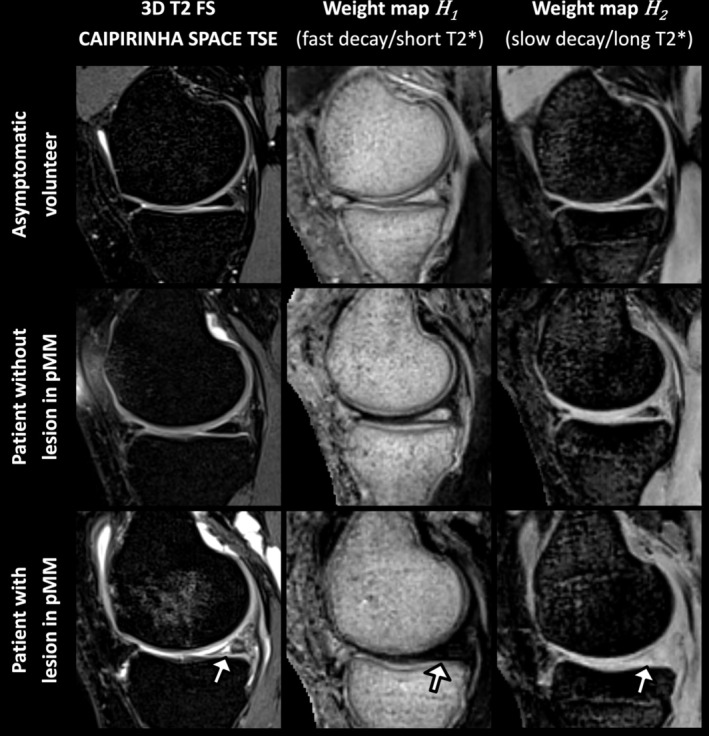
Sagittal 3D T_2_ FS CAIPIRINHA SPACE TSE images and weight maps $H_{1}$ and $H_{2}$, respectively corresponding to the fast‐ and slowly‐decaying characteristic signal components, of the left knee of a 23‐year‐old female asymptomatic volunteer (top row), the right knee of a 33‐year‐old female patient with sustained knee pain (middle row) and the left knee of a 72‐year‐old female patient reporting episodes of knee giving away (bottom row). No lesions were observed in the posterior horn of the medial meniscus (pMM) of the 33‐year‐old female patient on anatomical MR images, while a complex tear was noted in the pMM of the 72‐year‐old female patient (white arrows). The lesioned pMM showed lower weights (darkening) in the weight map $H_{1}$ and increased weights (brightening) in the weight map $H_{2}$ in comparison to the maps of the asymptomatic volunteer. For the female patient with no detected pMM lesion, a slight darkening in $H_{1}$ and shallow brightening of $H_{2}$ can be observed for the pMM region. Weight maps for the fat‐like component and the component related to the water–fat mixing (chemical shift) are not shown as the contributions of these components in the pMM region were negligible.
